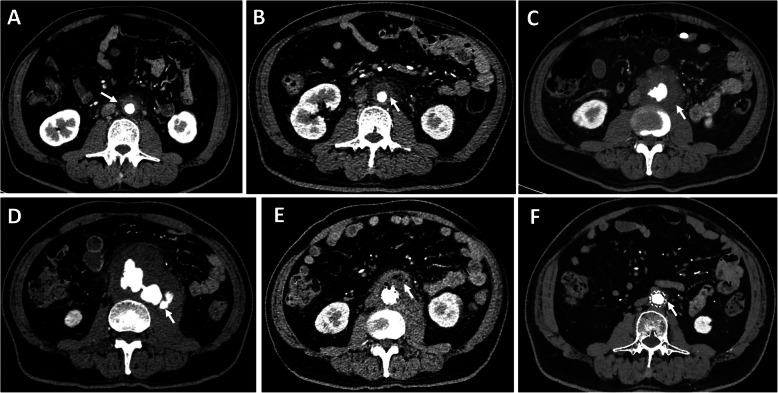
CTA showed the dynamic changes (arrow) of infrarenal abdominal aorta: a a periaortic fat stranding, b a hypo-attenuating rim, c irregular soft-tissue mass, d a lobulated pseudoaneurysm, e postoperative left psoas muscle abscess and periaortic abscess with gas collection, and f normal image. CTA = computed tomography angiography
